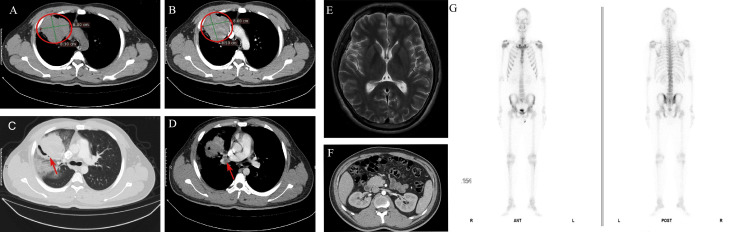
Imaging findings Chest CT scan (A, red circle, mediastinal window) and enhanced CT (B, red circle, mediastinal window) in axial planes demonstrate a large soft tissue mass measuring approximately 8.1 cm × 8.0 cm in the right upper lobe, accompanied by alveolar hemorrhage, partial bronchial obstruction (C, red arrow, lung window), and enlargement of right hilar lymph nodes (D, red arrow, mediastinal window). No lesions were identified in the brain (E, MRI), abdominal organs (F, contrast-enhanced CT), or bones (G, emission computed tomography (ECT)).
